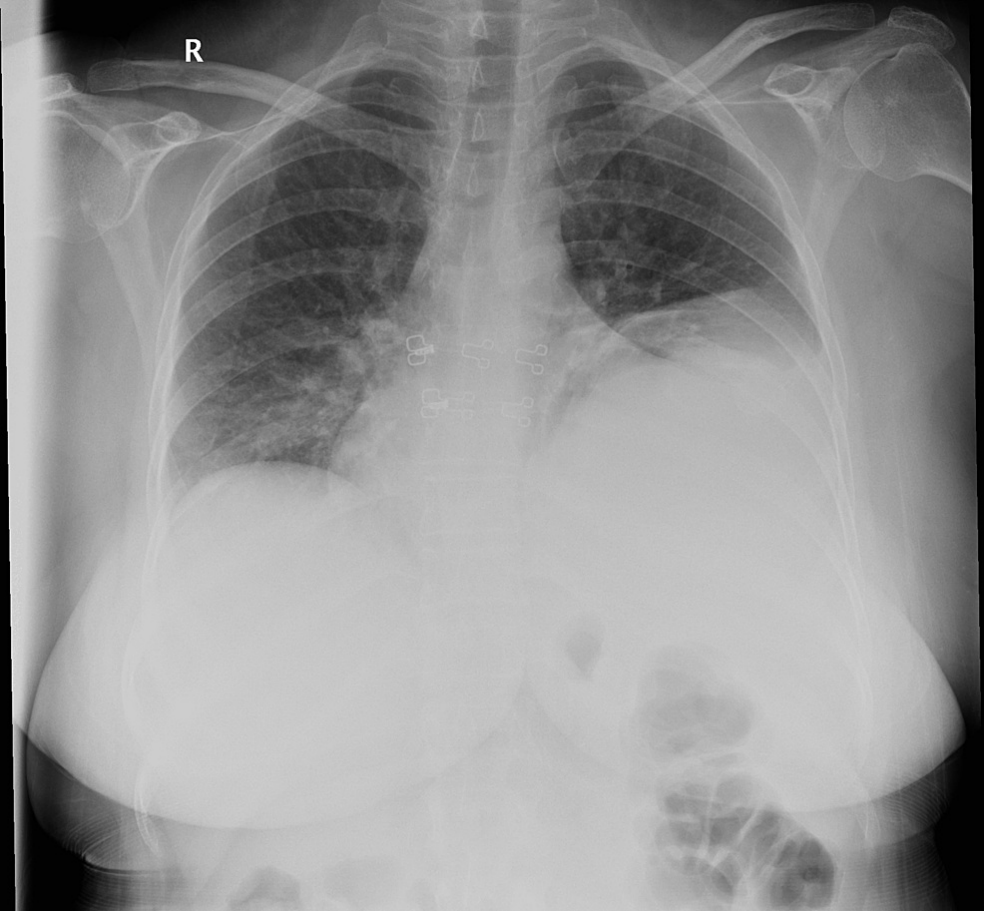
The chest x-ray displayed an opacity extending into the left costophrenic angle.
